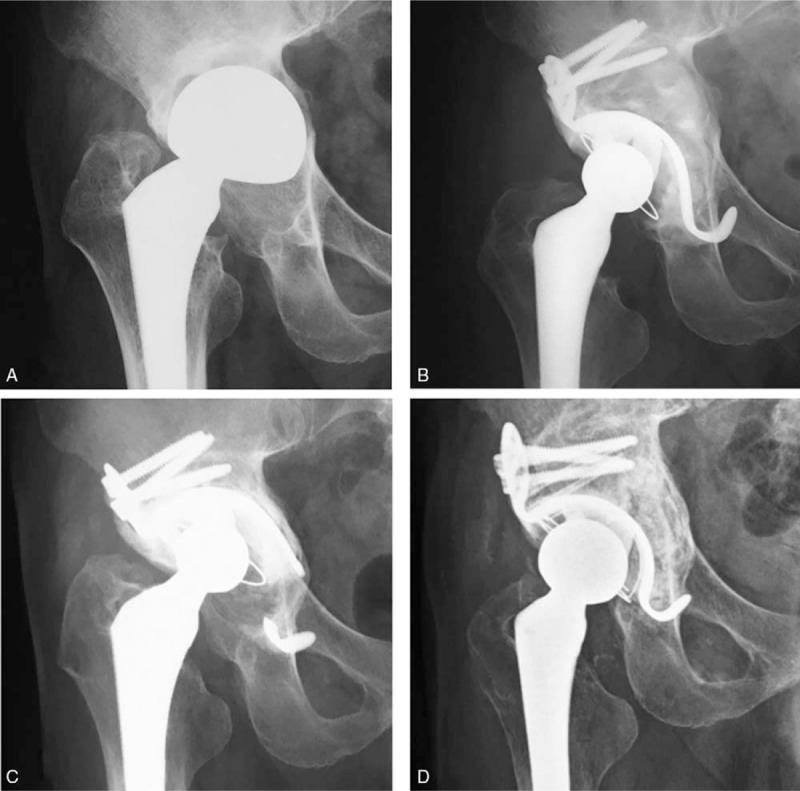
X-ray radiograph of the hip with 57-year-old man. A. at initial visit. B. after first revision surgery with HH-type KT and a structural bone graft. C. ten years after revision surgery. D. after re-revision surgery with OC-type KT and a structural bone graft. HH = high hip center-type, KT = Kerbull-type acetabular support rings, OC = original cup center-type.
